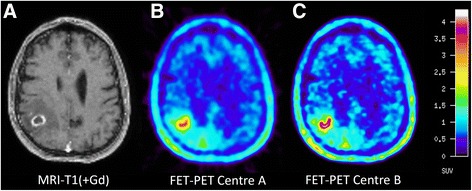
Glioblastoma in the right parietal lobe. Contrast-enhanced T1-weighted MRI (a) shows a ring-enhancing lesion. FET PET image based on the method of centre A (b) shows lower noise but the image based on the method of centre B (c) shows a sharper demarcation of the metabolically active tumour parts
